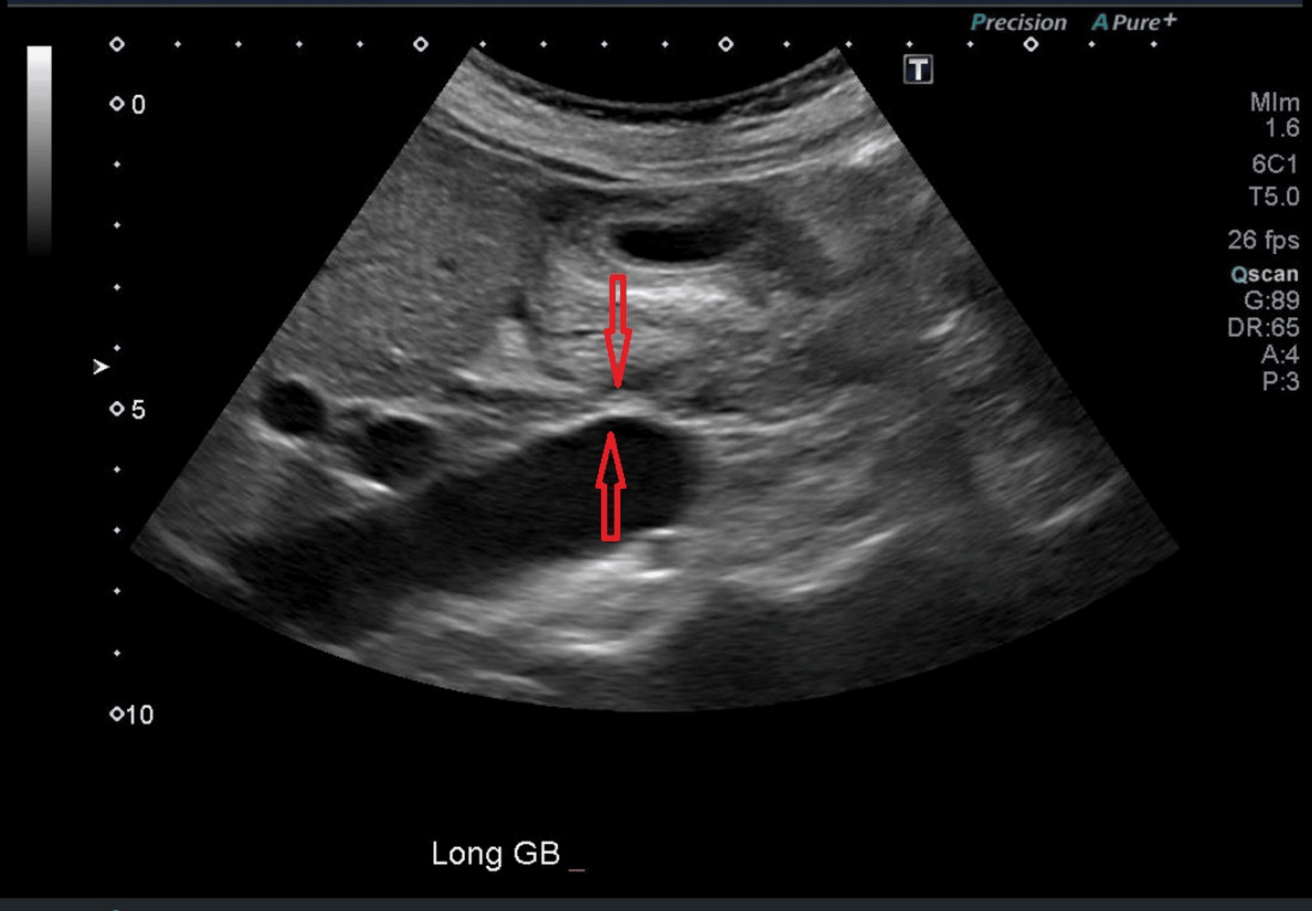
Abdominal ultrasound showing gallbladder wall thickening
